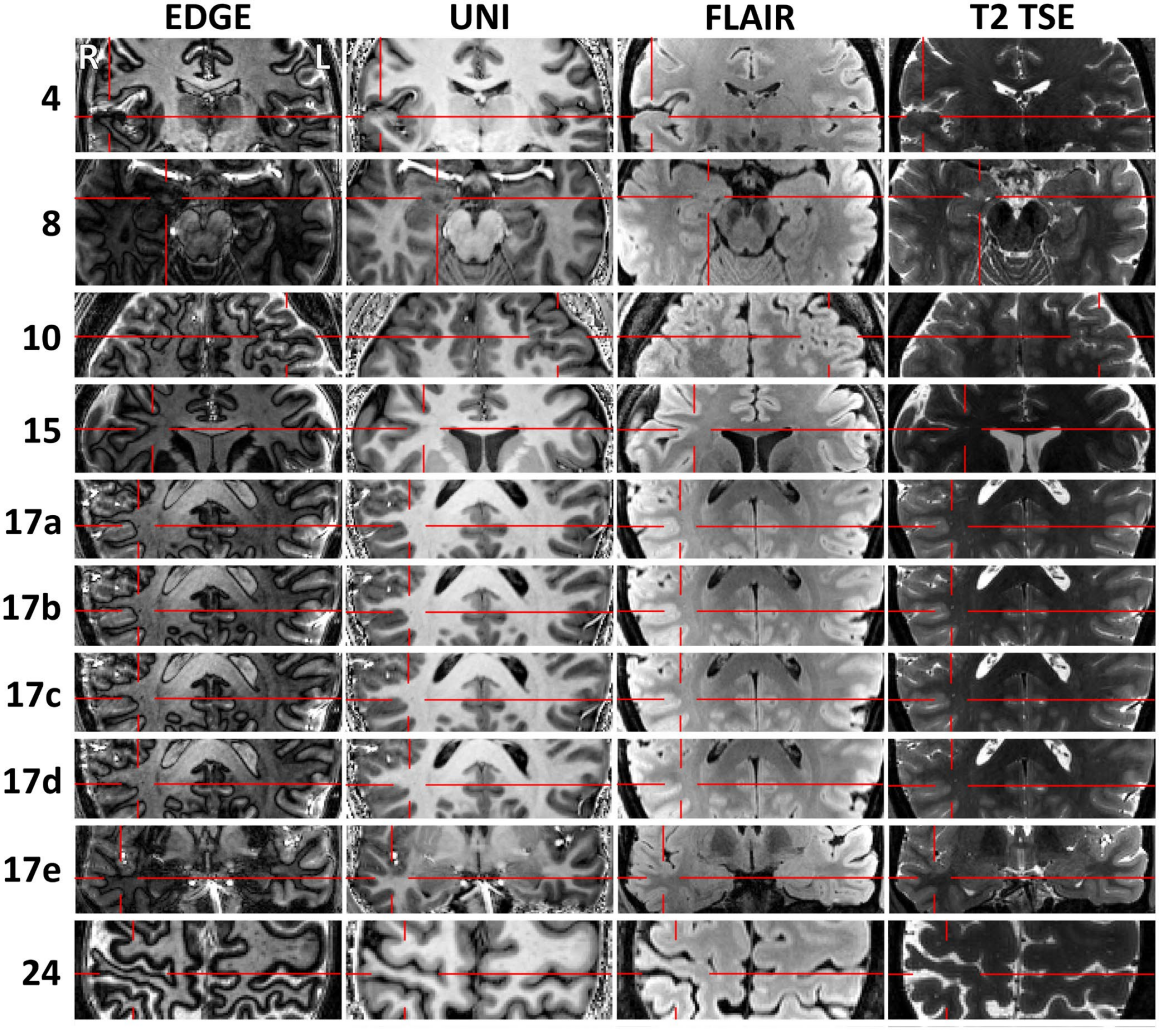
Putative lesions requiring further investigation before a surgical plan could be made. The putative lesions in: Patient 4 cortical–subcortical blurring of uncertain significance, stereo‐electroencephalography (sEEG) planned to evaluate significance; Patient 8 likely amygdala lesion, sEEG planned to evaluate significance; Patient 10 extensive polymicrogyria, sEEG planned to narrow down the epileptogenic zone; Patient 15 likely FCD, ictal SPECT requested for confirmation; Patient 17 rows a–d show four consecutive slices revealing that an equivocal lesion on 3T magnetic resonance imaging (MRI) is in fact a vessel, whereas row e shows a new possible FCD awaiting sEEG evaluation; Patient 24: Gray matter thinning likely representing perinatal infarction; sEEG planned to narrow down the epileptogenic zone.
